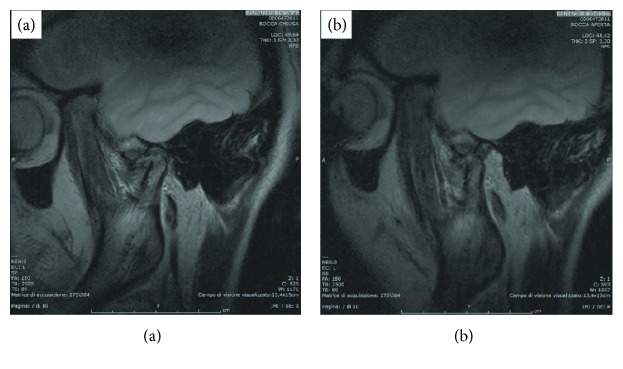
MRI at the five-month follow-up. Closed (a) and open mouth position (b) showing the HAM patch. The HAM patch covers the condyle head; the translation is improved.
